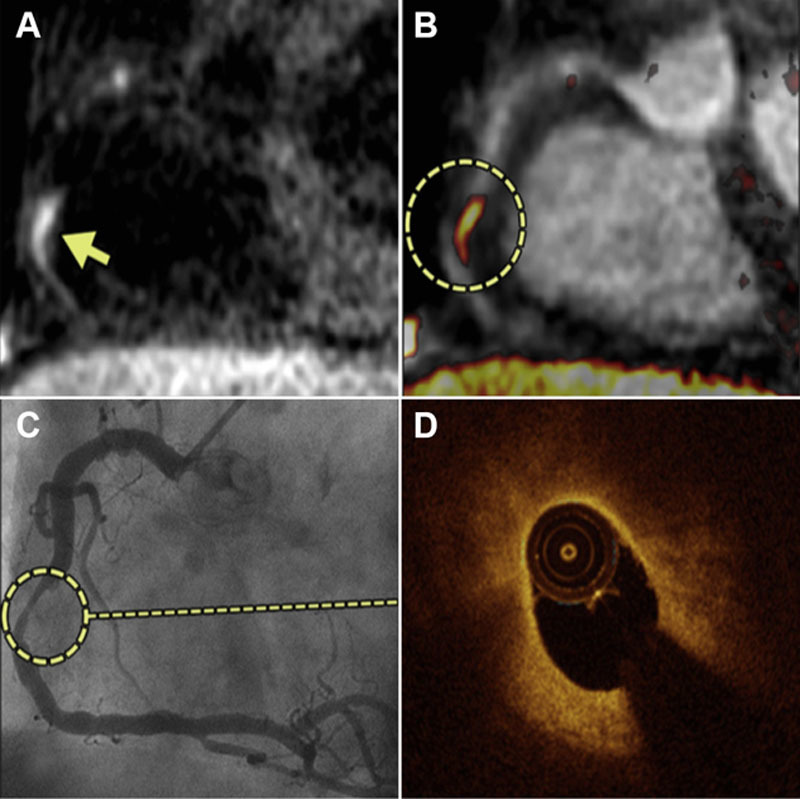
Hyperintense coronary signal on T1-weighted magnetic resonance image (MRI). A, T1-weighted MRI showing hyperintense signal within the wall of the right coronary artery (arrow), highlighted by fused MRI (circle, B); C, X-ray angiography shows severe stenosis in corresponding coronary segment, with lipid-rich plaque in this same region on optical coherence tomography (OCT) (D). Adapted from Matsumoto et al^78^ with permission of the publisher. Copyright ©2015, Elsevier.
